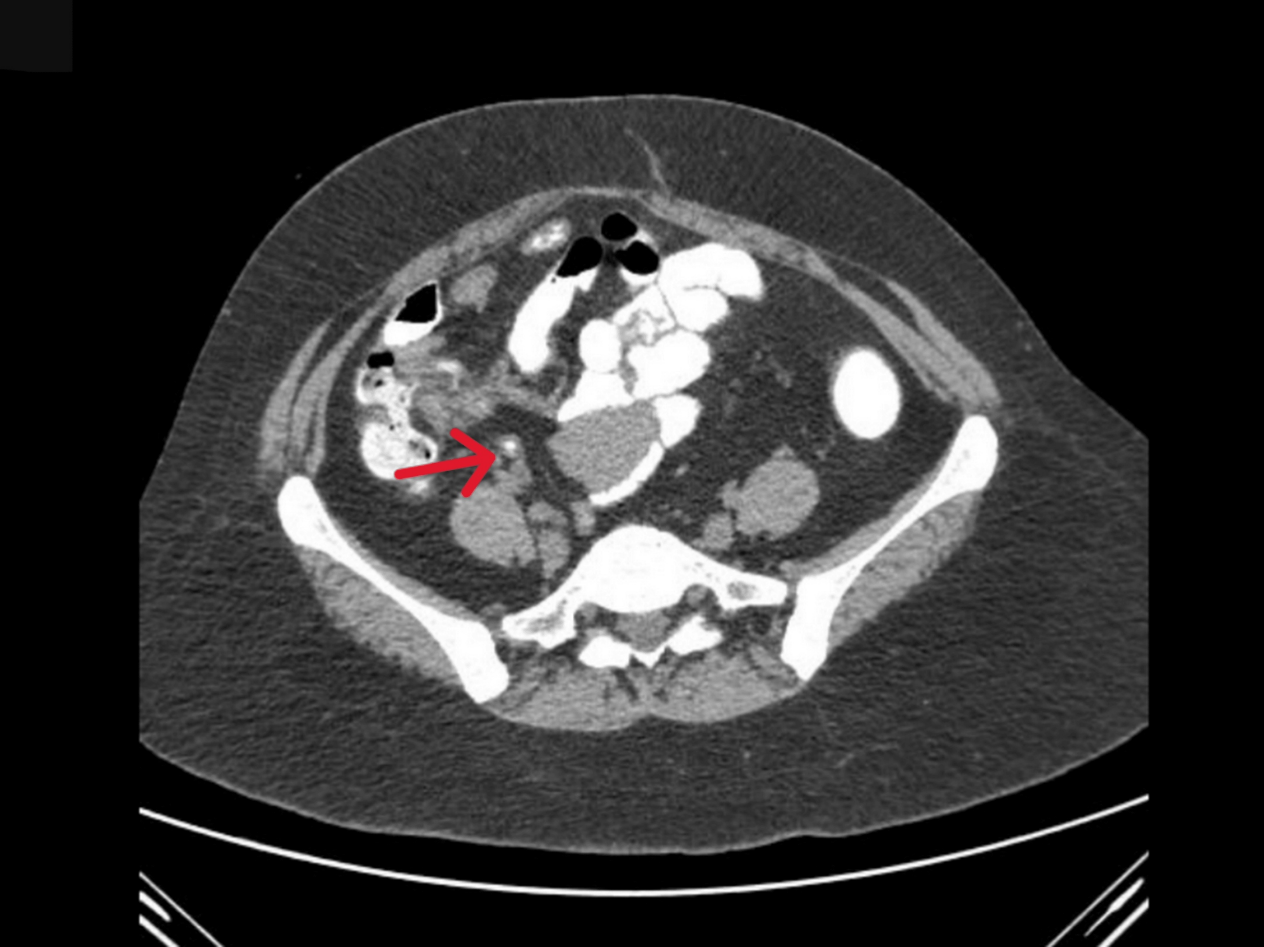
Contrast-Enhanced CT Showing an Appendicolith in Acute Appendicitis (Case 2) Axial CECT image reveals a distended appendix containing a hyperdense intraluminal appendicolith (red arrow), with periappendiceal fat stranding and small reactive mesenteric lymph nodes. These findings are typical of acute appendicitis with luminal obstruction due to a fecalith.
